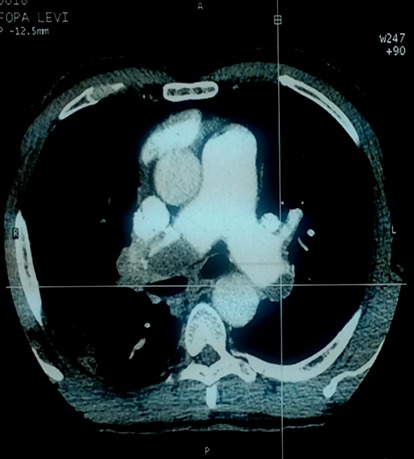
Thoracic CT scan showing thrombus in the right and left branches of the pulmonary arteries
